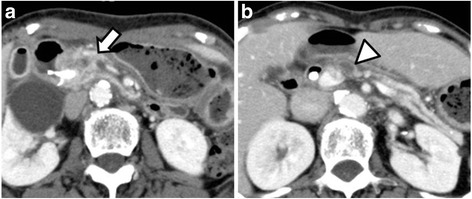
CT images after chemoradiation therapy (a, b)
